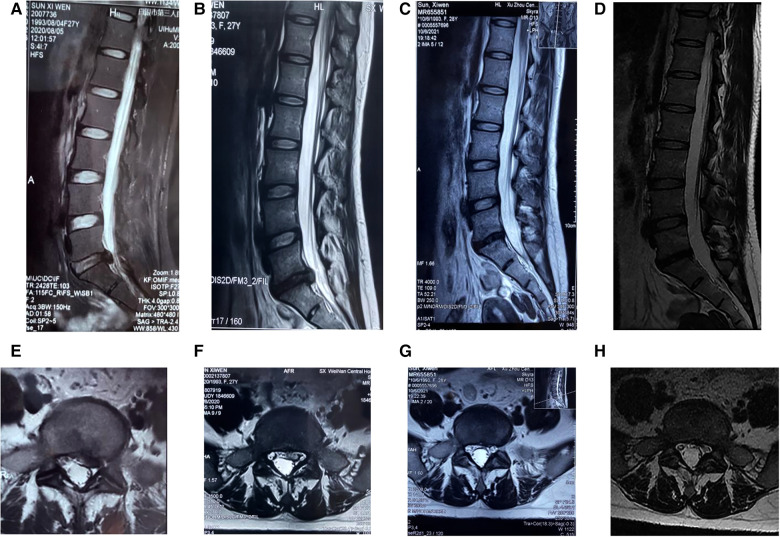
MRI of a 27-year-old woman with lumbar disc herniation with L5/S1 left center prolapse who was treated with full endoscopic nucleus pulposus resection combined with annulus fibrosus suture. (A,E) Preoperative sagittal and horizontal MRI showing grade III disc degeneration, compression and deformation of the dural sac, and compression of the right nerve root. (B,F) MRI at 3 months postoperatively shows the absence of prominent nucleus pulposus, no compression of the dura, and no obvious stenosis of the lateral recess. (C,G) MRI at 1 year postoperatively shows no significant disc herniation. (D,H) MRI at 18 months postoperatively shows the same as at 1 year.
